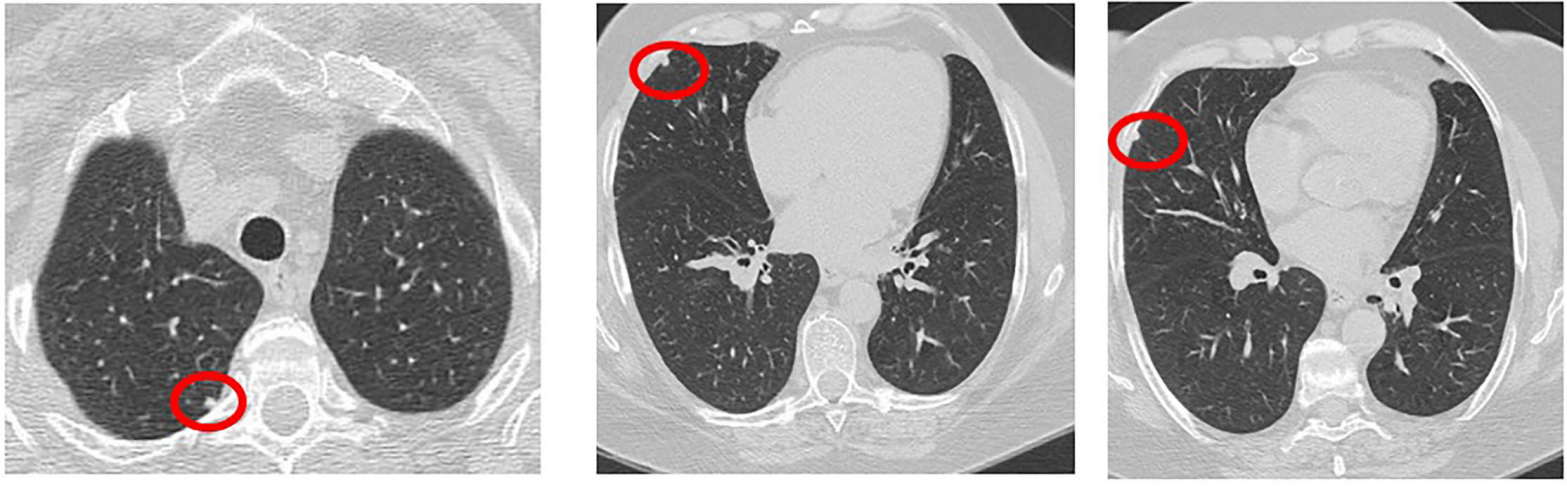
Multiple pulmonary nodules on HRCT in a patient with RA.
